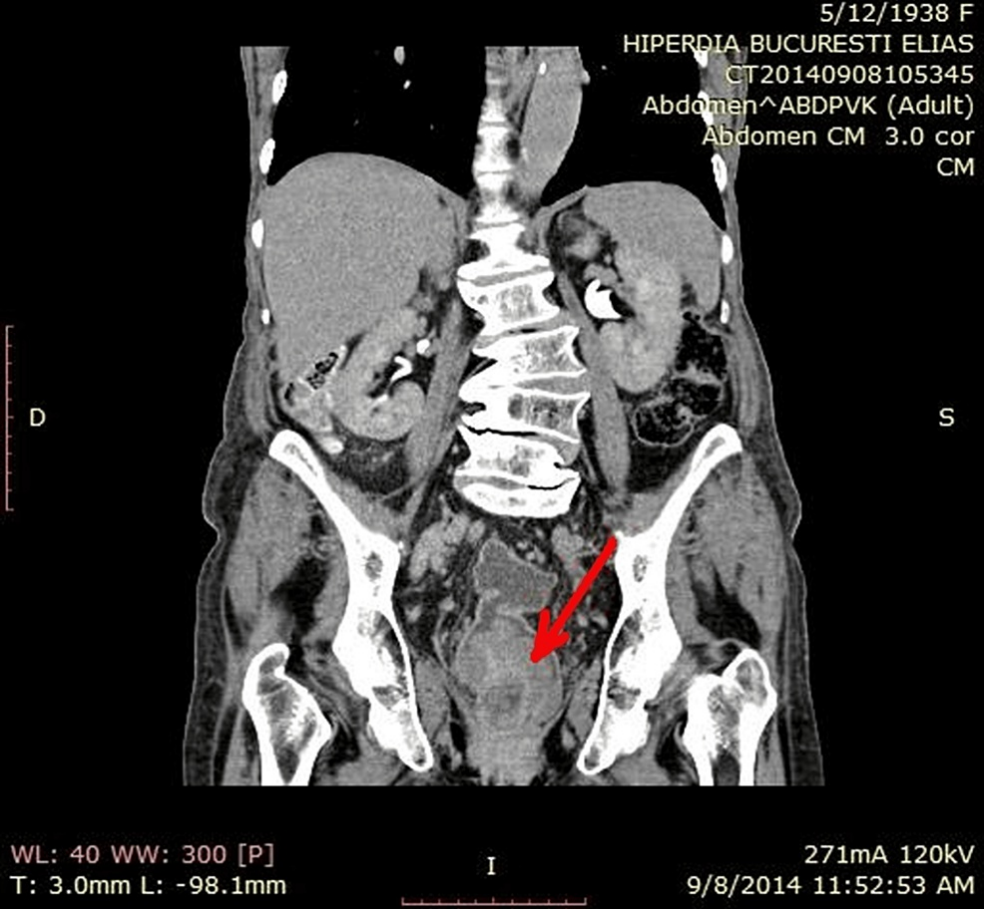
Computed tomography scan of the anal-rectosigmoid mass Coronal view showing the anal-rectosigmoid mass measuring 6.8 cm × 5.3 cm, with invasion into perirectal structures and multiple local-regional adenopathies. Degenerative disc and vertebral changes are highlighted with arrows indicating the tumor location.
